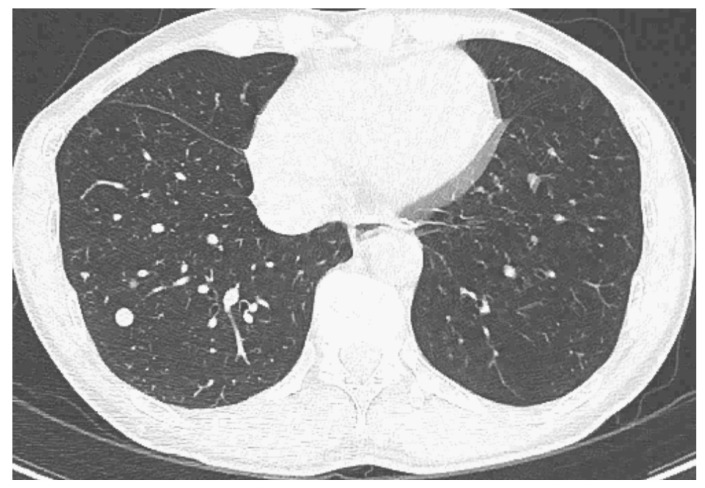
Pre-treatment chest CT with enhancement, showing multiple lung metastases.
